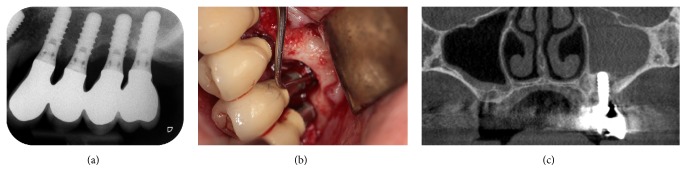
Ten years after implant placement. (a) Periapical radiograph, (b) clinical view, flap at site 25, and (c) cone-beam computed tomography image. Note the opacity of the left sinus.
